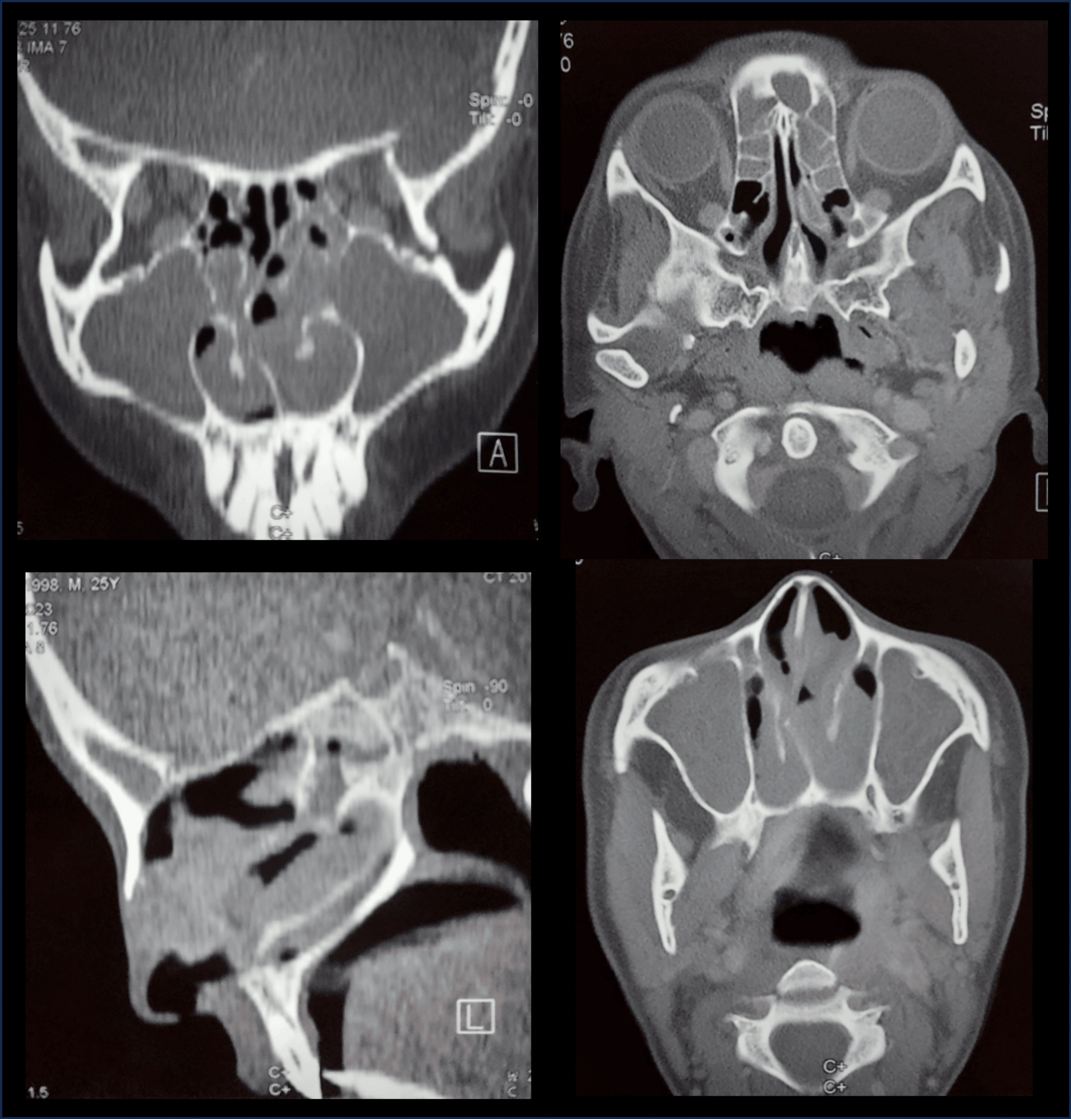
Sinus CT scan showed nasal polyposis.
